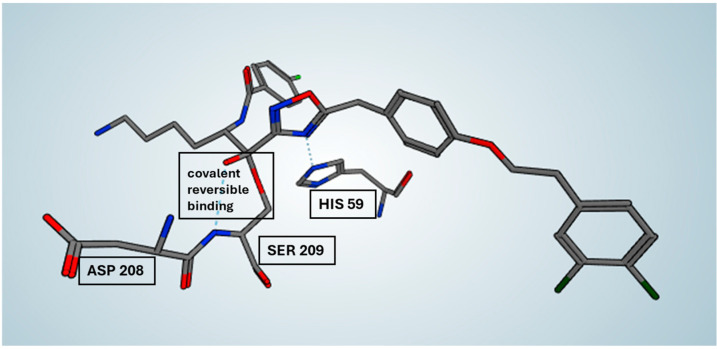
Focus on the reversible covalent binding mode of compound #1 (violet) in the X-ray complex structure with tryptase.
